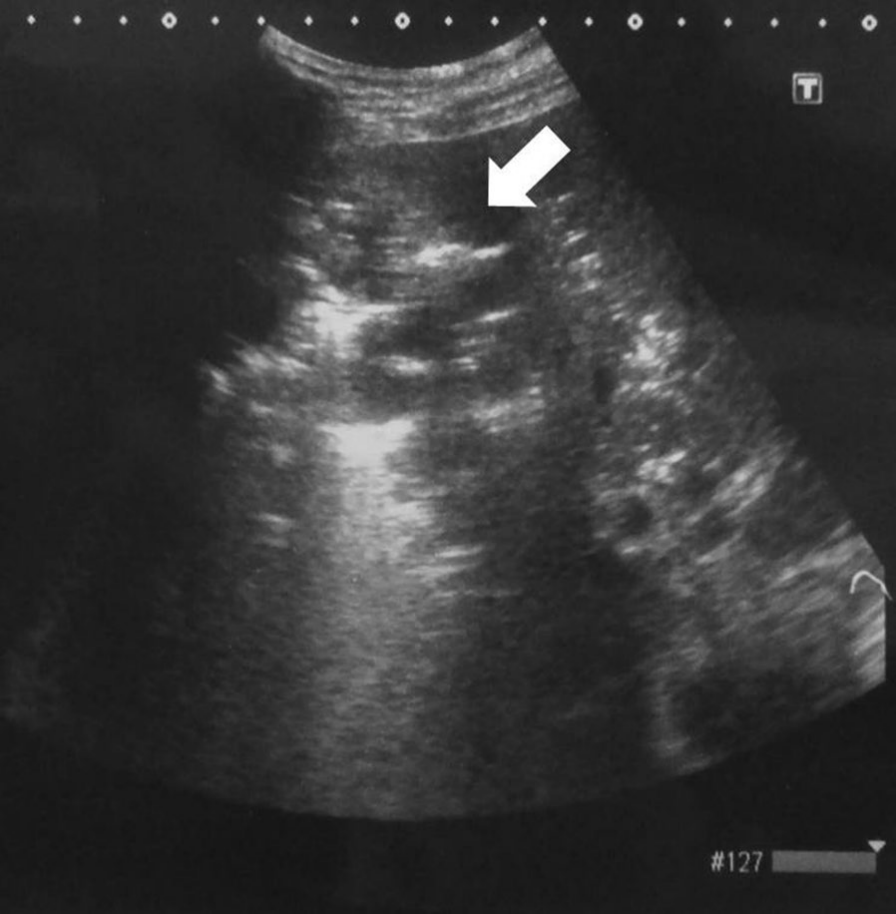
A hypoechoic lesion with indistinct boundaries was observed in the right hepatic lobe on abdominal ultrasound, and several hyperechoic findings suggestive of air were observed within this lesion (*arrow*)
